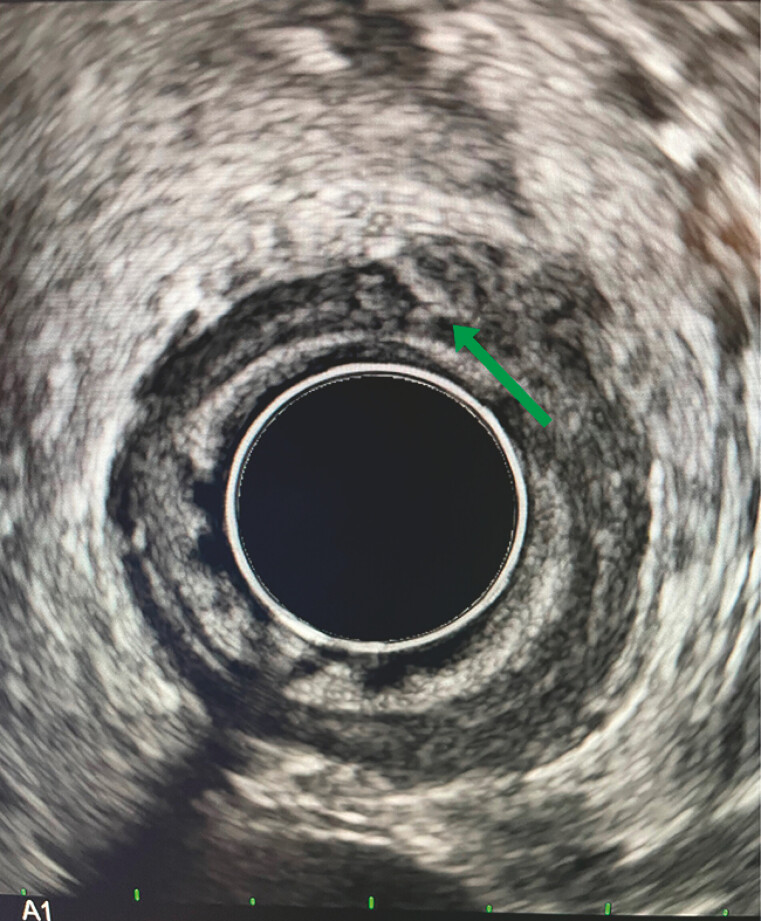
Endoscopic ultrasound showed a linear hyperdensity projecting through the muscularis propria.
